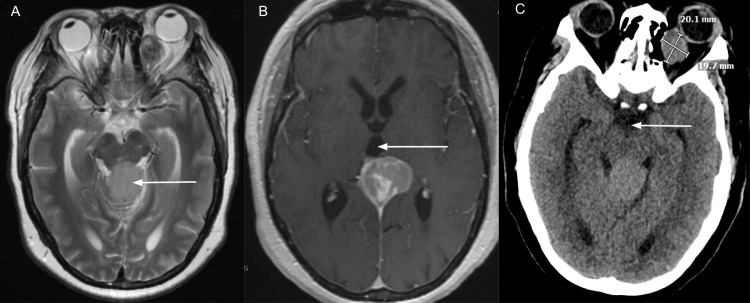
Preoperative MRI and CT images depicting the pineal mass and mass effect on surrounding tissues. A: T2-weighted magnetic resonance imaging (MRI) with arrow depicting pineal gland tumor compressing dorsal midbrain, B: T1-weighted MRI with arrow depicting dilated third ventricle, C: Non-contrast computed tomography of the brain showing cerebrospinal fluid space around the midbrain.
